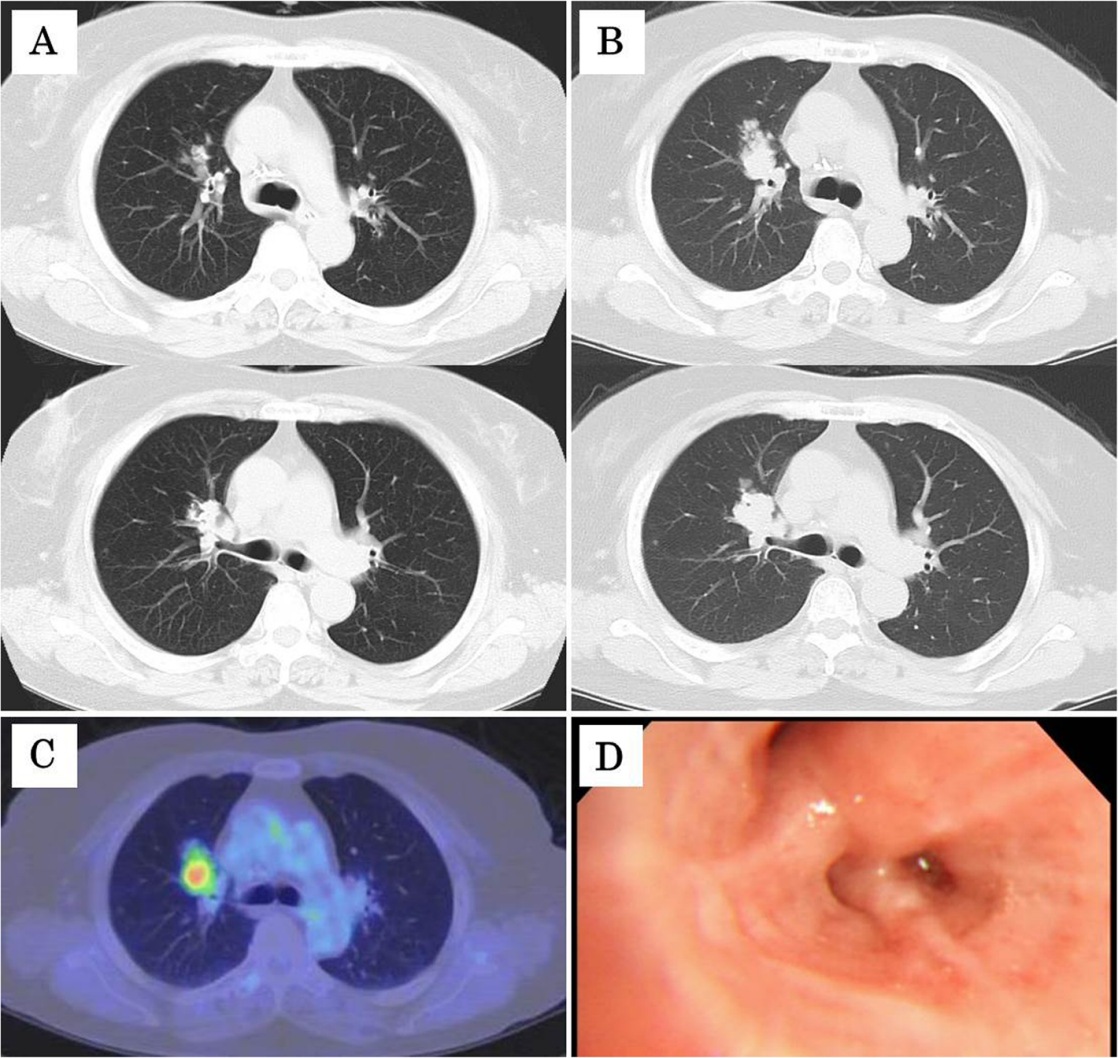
a Original CT scan obtained 3 years ago showed a pulmonary nodule in the upper area of the right lung. b CT scan on admission showed an enlarged mass. c FDG-PET indicated high accumulation in the mass region (SUV max = 6.5). d Bronchoscopic examination revealed narrowing of the superior bronchial ostia. The bronchial mucosa was macroscopically normal
